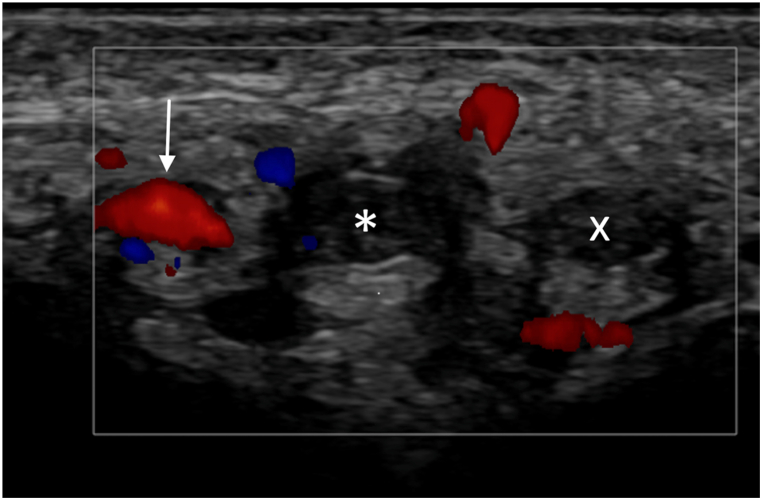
Doppler ultrasound image acquired using a 15MHz linear ultrasound probe showing hyperechoic blood clot within the dorsal penile vein (white star) and the left dorsal penile artery (white cross). There is a persistent doppler signal in the right dorsal penile artery (white arrow).
